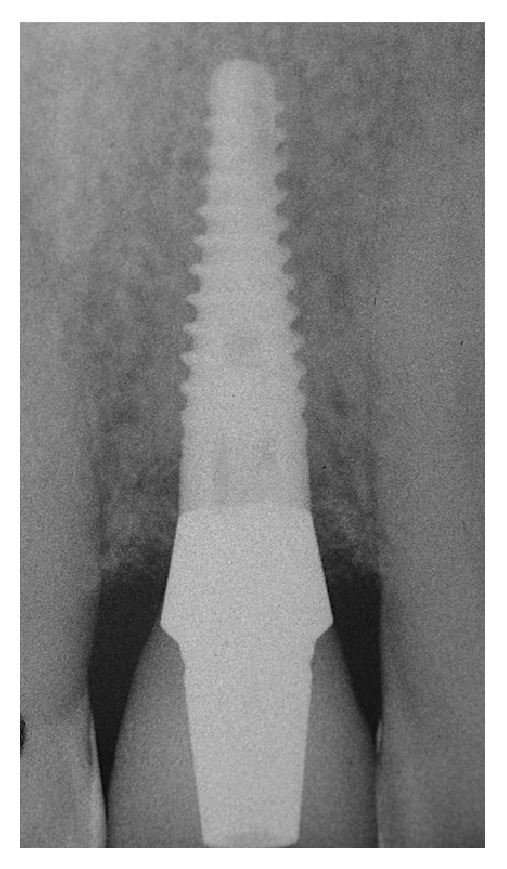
The periapical radiograph of the implant and the screw-retained restoration (one piece) 38 months after implant loading. Almost no edgeal bone loss was noted around the implant.
